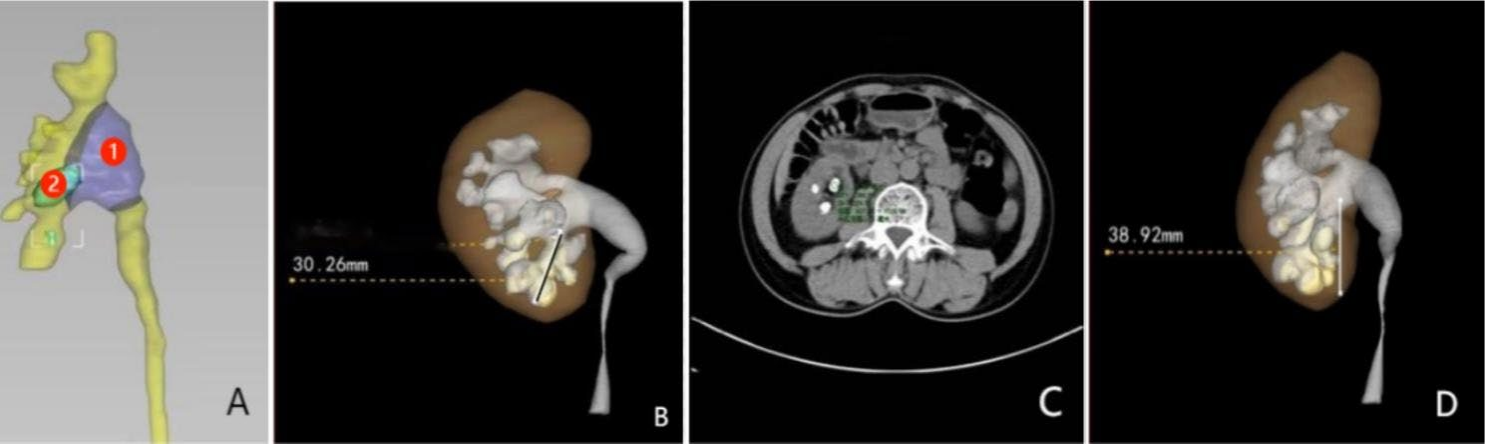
(A) VR imaging and measurement. 1: Renal pelvis volume (one end is the junction of the renal pelvis and ureter; the other end is the portion of the renal pelvis except for renal calyx). 2: Stone surface area. (B) Length of the calyces funnel (distance between the farthest point of the lower calyx where the calculus is located and the middle point of the lower lip of the renal pelvis). (C) Essence of stone (average CT value of the calculus). (D) Pelvic calyceal height (vertical distance between the horizontal line of the lowest point of the lower calyces where the calculus is located and the highest point of the lower lip)
